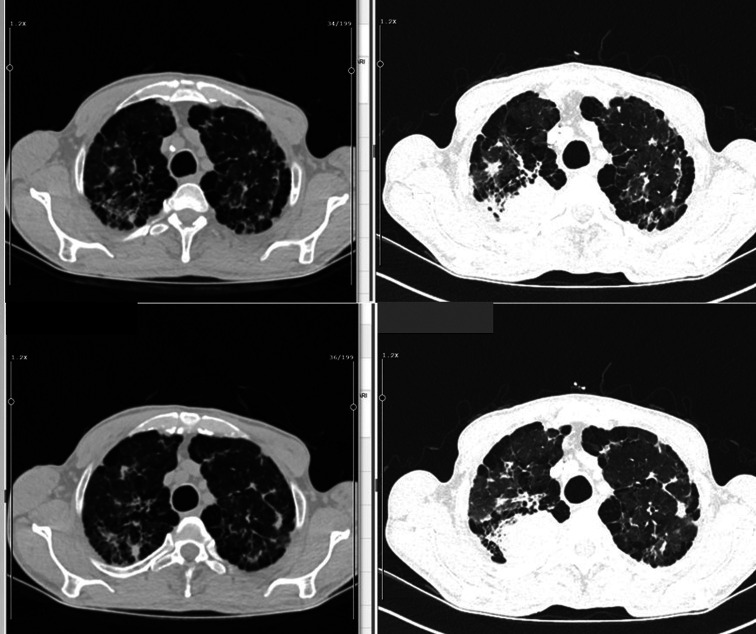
(A) Chest computed tomography taken 23 months prior (left side) (B) at the time of diagnosis (right side).
